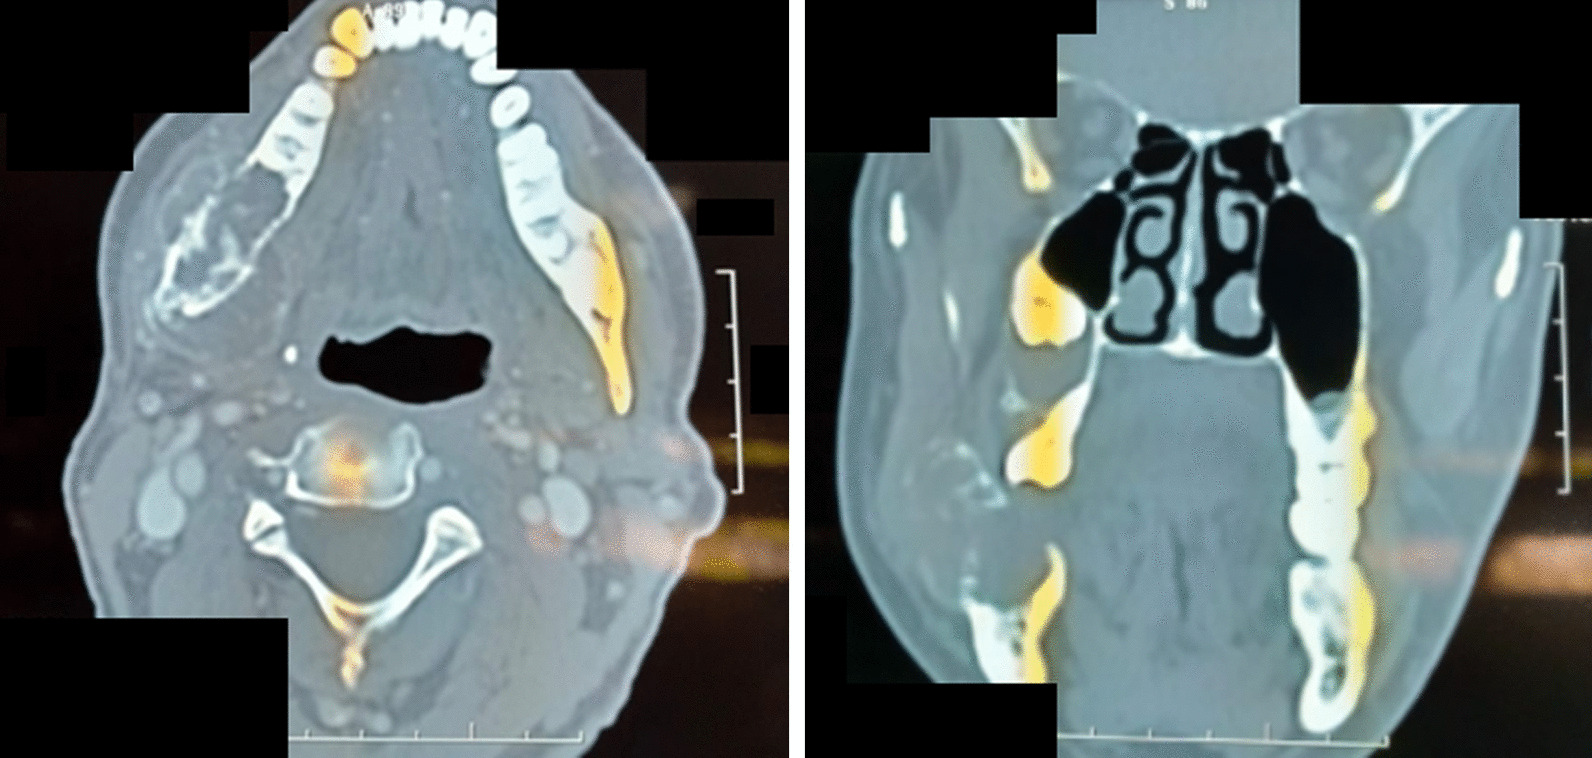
Computed tomography scan showing a large osteolytic spontaneously hypointense and multilobulated lesion associated with calcified areas with extension to the masseter muscle and the anterior belly of digastric muscle
